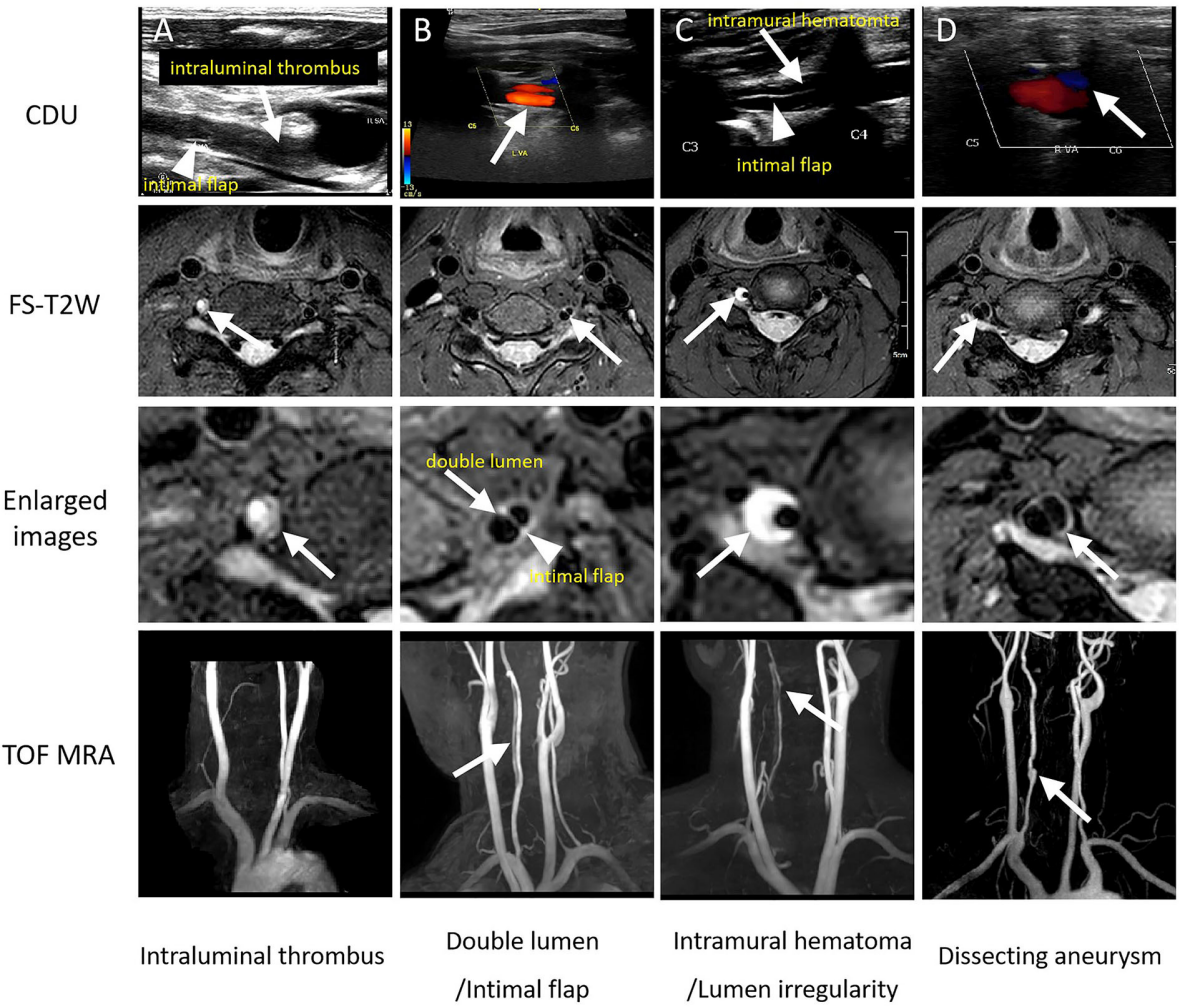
Characteristics of the extracranial vertebral artery dissection on color duplex ultrasonography (CDU) and its corresponding, pathognomonic high-resolution magnetic resonance imaging signs. The (A–D) four columns represent four patients (Patients # 1, 2, 3, and 4), respectively, and each column from top to bottom is CDU, fat-suppressed T2-weighted (FS-T2W), magnified view of FS-T2W, and 3D time-of-flight magnetic resonance angiography (TOF) imaging.
